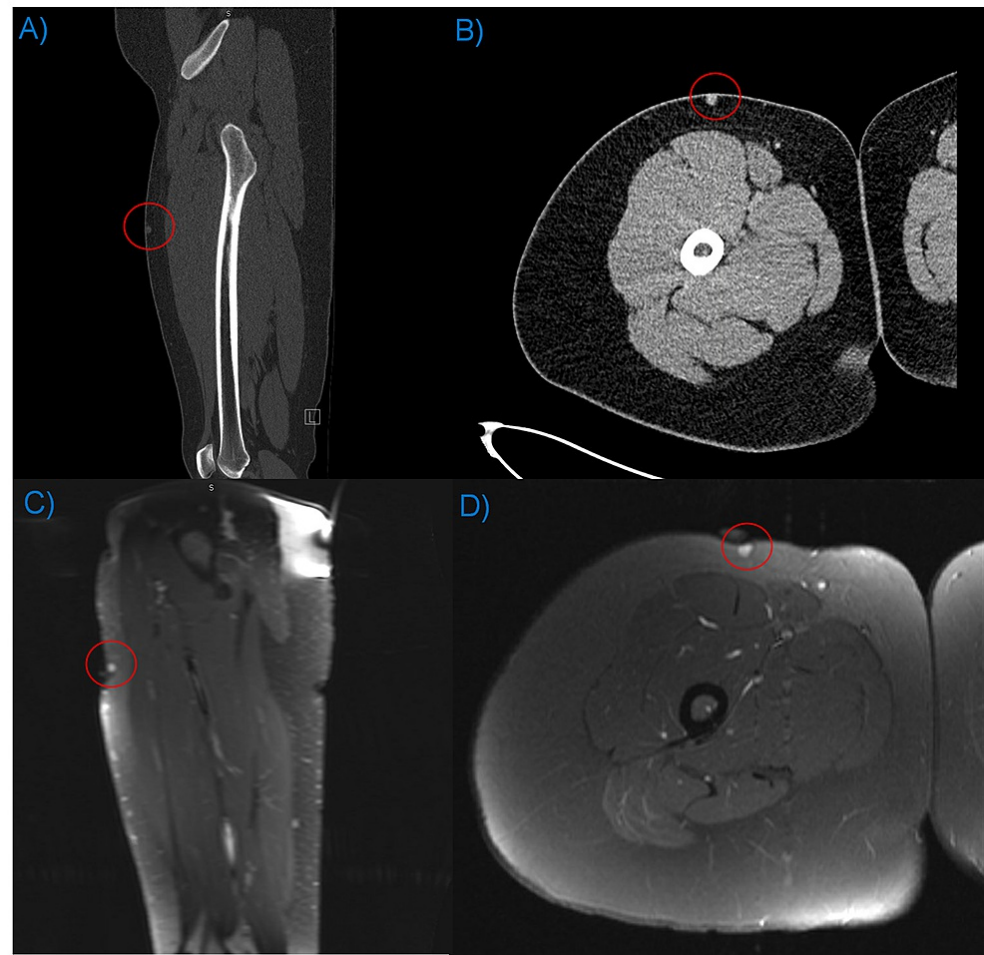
Pre-operative CT and MRI. Contrasted CT and MRI of the right lower extremity; A) sagittal contrasted CT, B) axial contrasted CT, C) sagittal T1 post-contrast MRI, D) axial T1 post-contrast MRI
